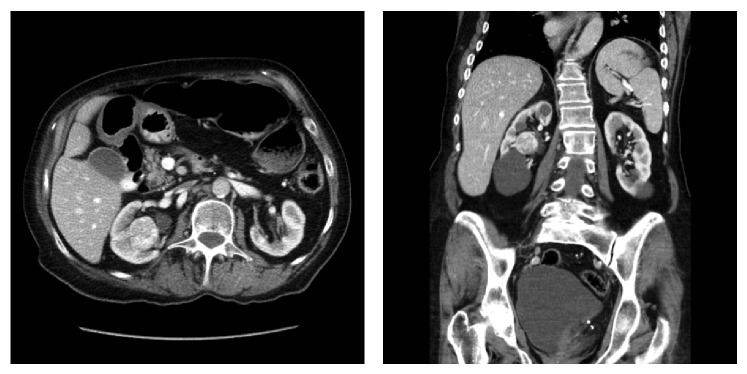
Axial and coronal infused CT imaging of patient 3.
